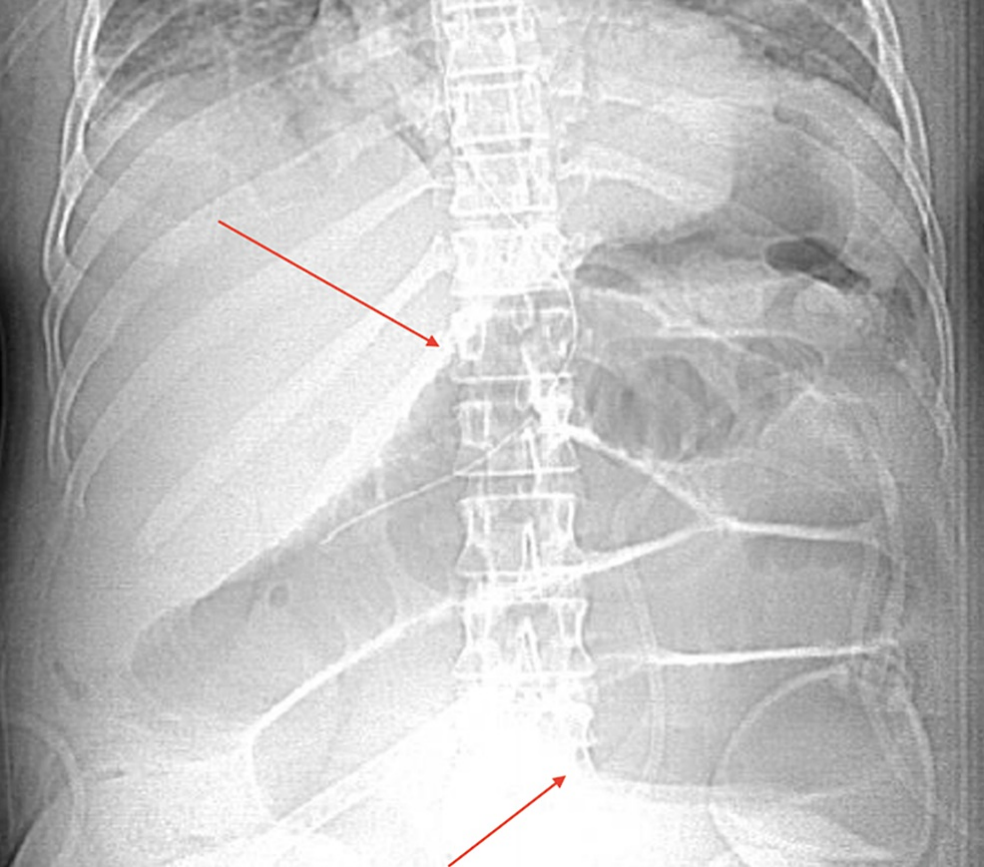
Abdominal x-ray indicating a postoperative ileus (red arrows)
